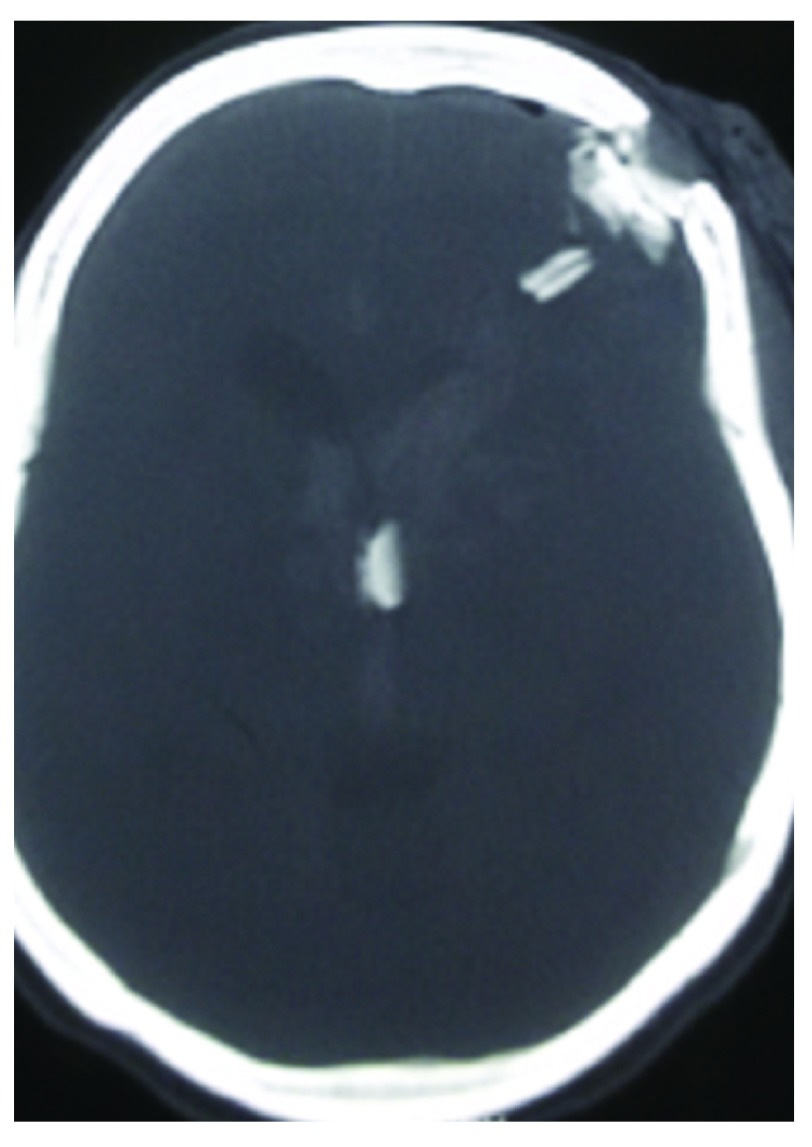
CT image showing the bone fragment lodged in the third ventricle.
